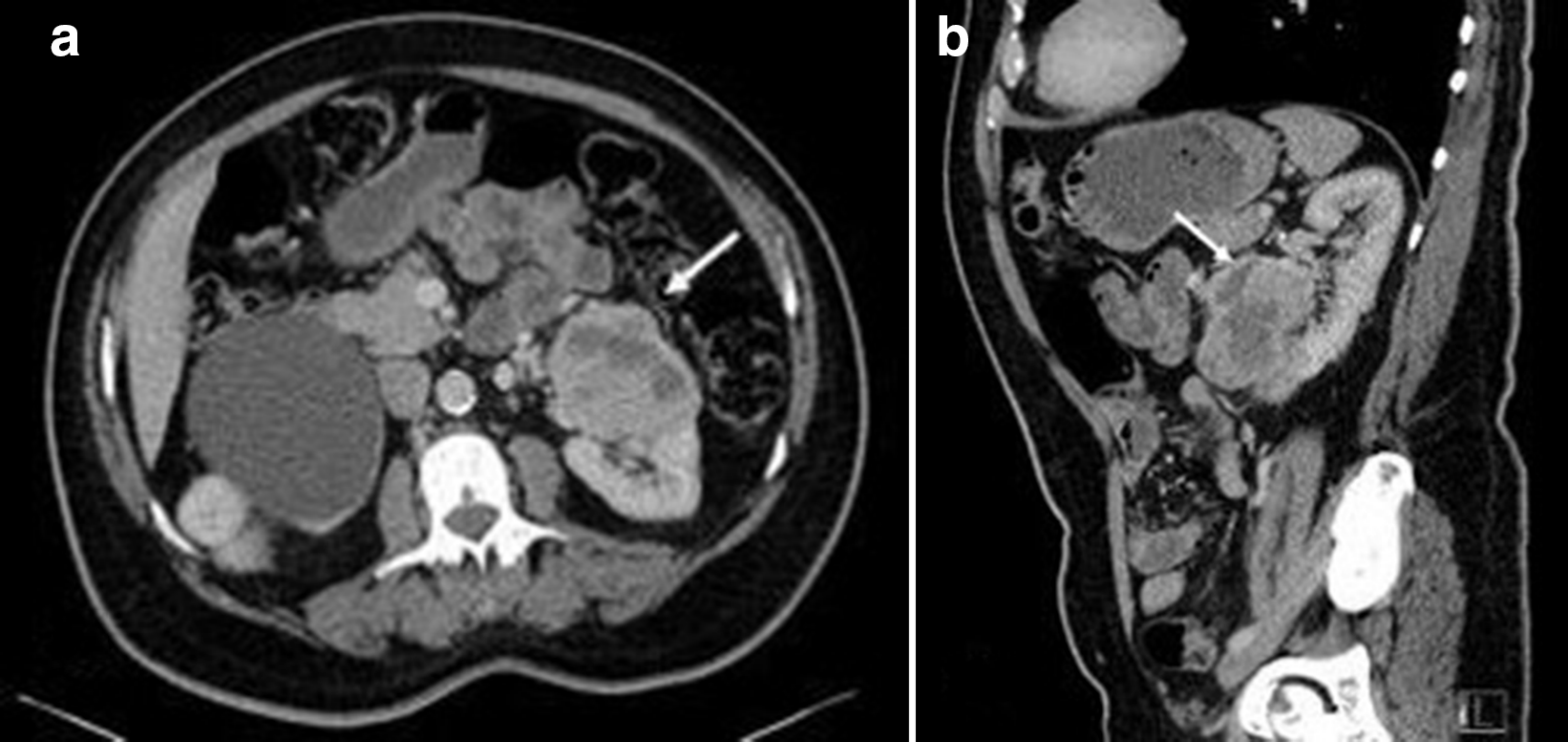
Axial (1a) and sagittal (1b) slices of CT abdomen and pelvis demonstrating
